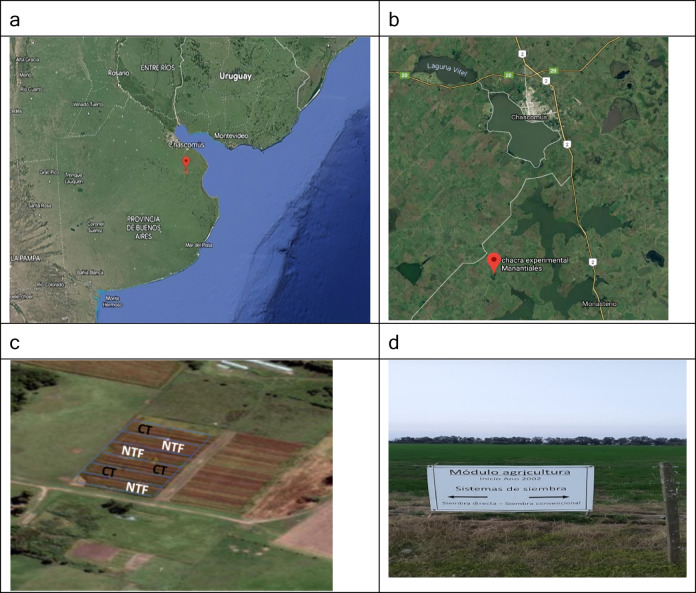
(a) Source: Google (n.d.). Chascomús, Buenos Aires, Argentina [Map]. Google Maps. Retrieved September 17, 2025, from (https://www.google.com/maps/place/Chascomús,+Provincia+de+Buenos+Aires). (b) Source: Google (n.d.). Chacra Integrated Experimental Chascomús (CEICh) [Map]. Google Maps. Retrieved September 17, 2025, from (https://www.google.com/maps/place/35°44’36.7"S+58°03’25.4"W). (c) Source: Google (n.d.). No-Till Farming (NTF) vs. Conventional Tillage (CT) trial. -35°44′38.2″S 58°03′11.1″W [Map]. Google Maps. Retrieved September 17, 2025, from (https://www.google.com/maps/place/35°44’38.2"S+58°03’11.1"W). (d) View of the experimental plot. Photograph taken by Maximiliano Gortari on August 25, 2022.
